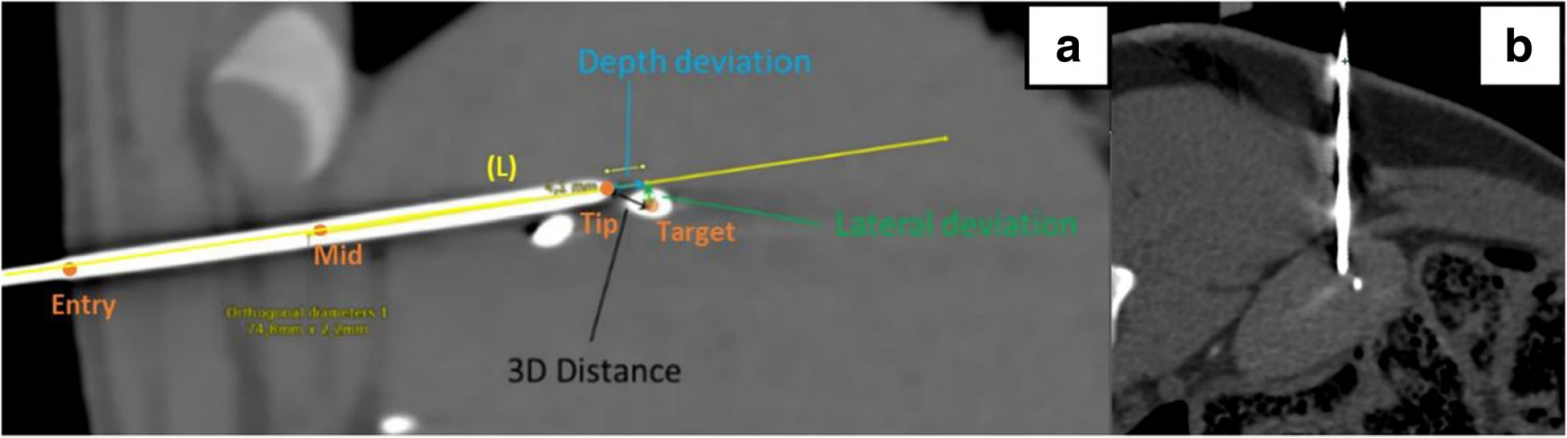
Technique of evaluation of accuracy based on a CT obtained after needle insertion. Reconstruction along the needle axis in the plane of the centre of the targeted fiducial is used for measurement of depth deviation, lateral deviation and three-dimensional distance (a). Typical multiplanar reconstruction along needle axis in the fiducial plane for evaluation of needle placement accuracy (b)
